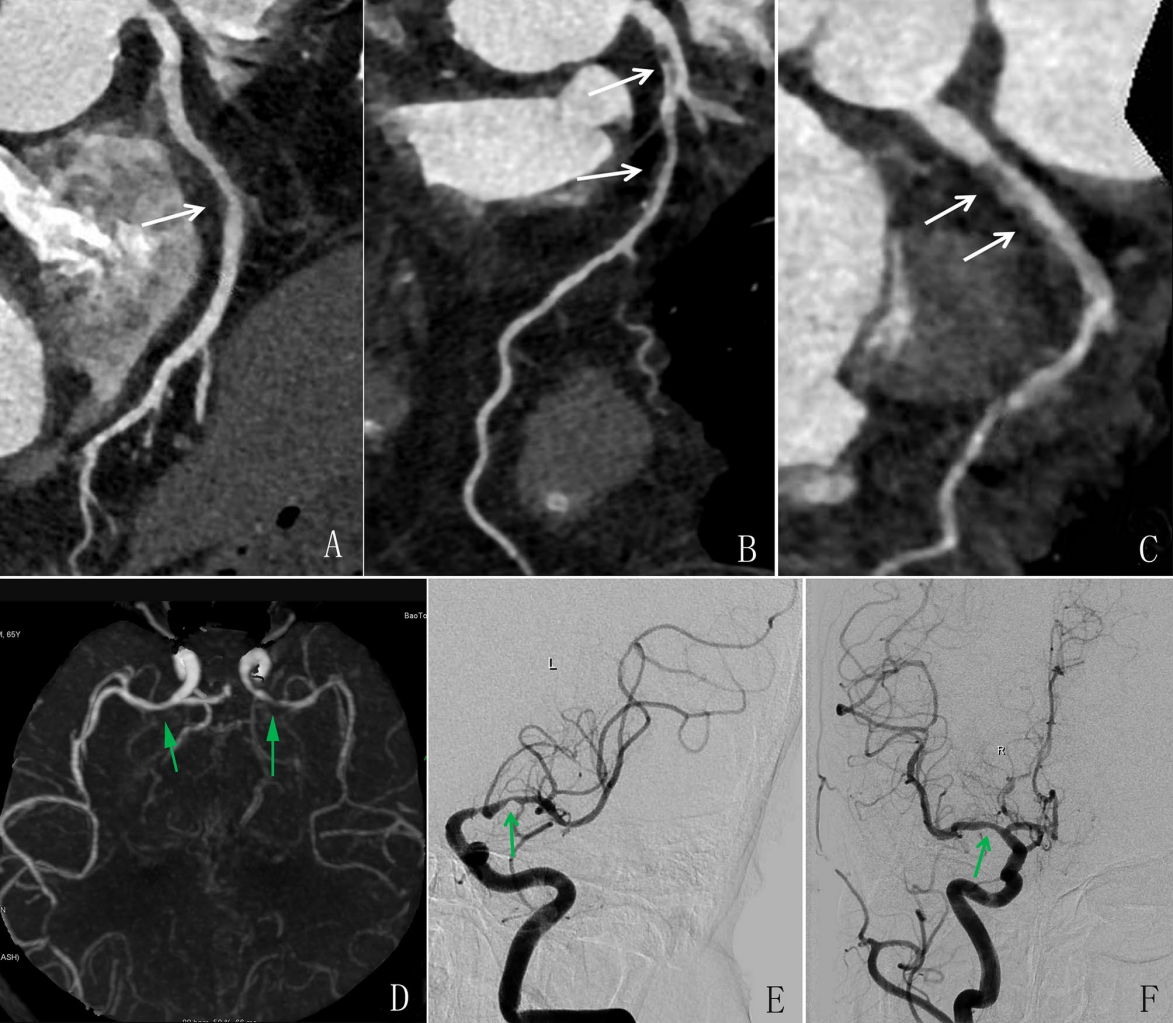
ICCC-CTA in a 72-year-old female patient with a 10-year history of diabetes. CPR of the RCA (A), LAD artery (B), and LCx artery (C) showed mild-to-severe stenosis with non-calcified plaques (*white arrow*). MIP of the cerebrovascular CTA showed mild stenosis in the M1 segment of the right MCA and severe stenosis in the M1 segment of the left MCA (*green arrow*). Invasive angiography confirmed stenosis in the left MCA (E) and right MCA (F) (*green arrow*). CPR, Curved planar reformation; ICCC-CTA, integrated coronary–carotid–cerebral computed tomography angiography; LAD, left anterior descending; LCx, left circumflex; MCA, middle cerebral artery; MIP, maximum intensity projection; RCA, right coronary artery
